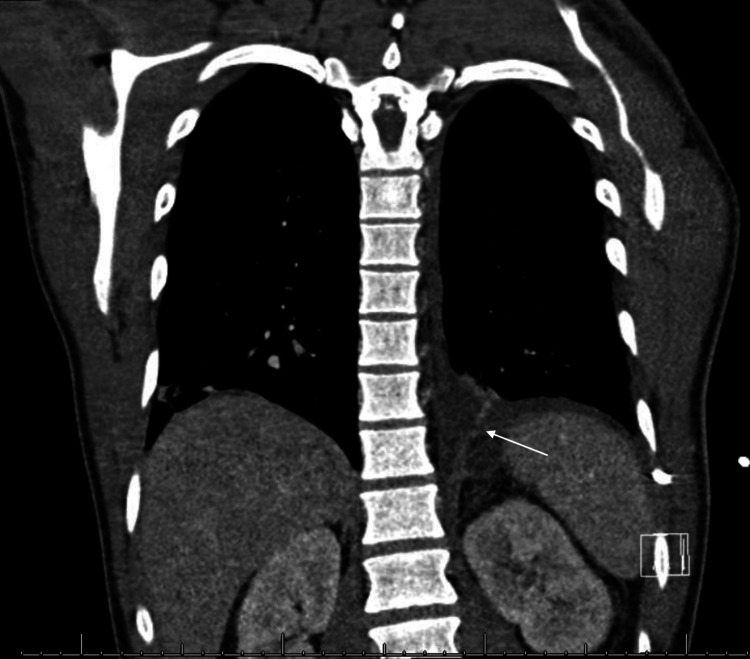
Post-thoracentesis chest CT with intravenous contrast demonstrating a probable communicating fistula between the sub-diaphragmatic and left pleural spaces (white arrow).
